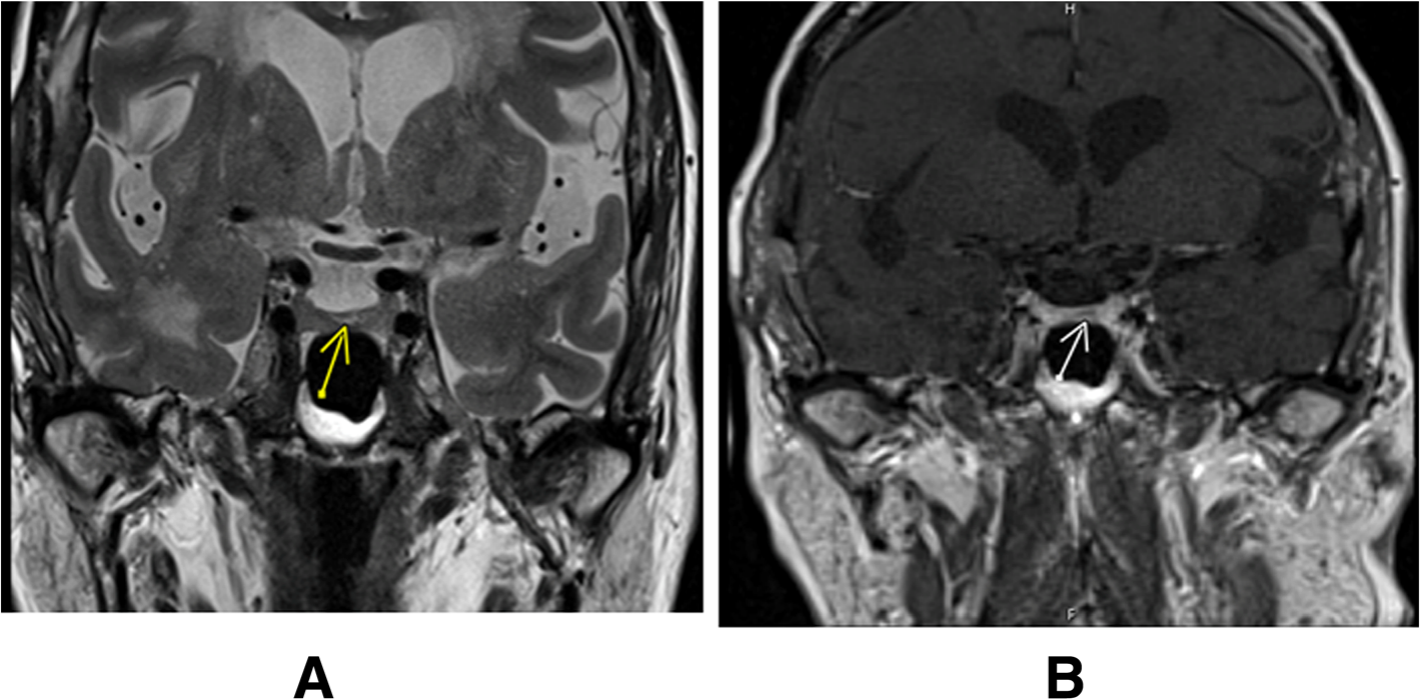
Preoperative MRI showing small pituitary microadenoma. Legend: Coronal MRI views show altered signal intensity within adenohypophysis T2 weighted sequence (Panel a), a region of delayed enhancement on the dynamic sequences (Panel b)
